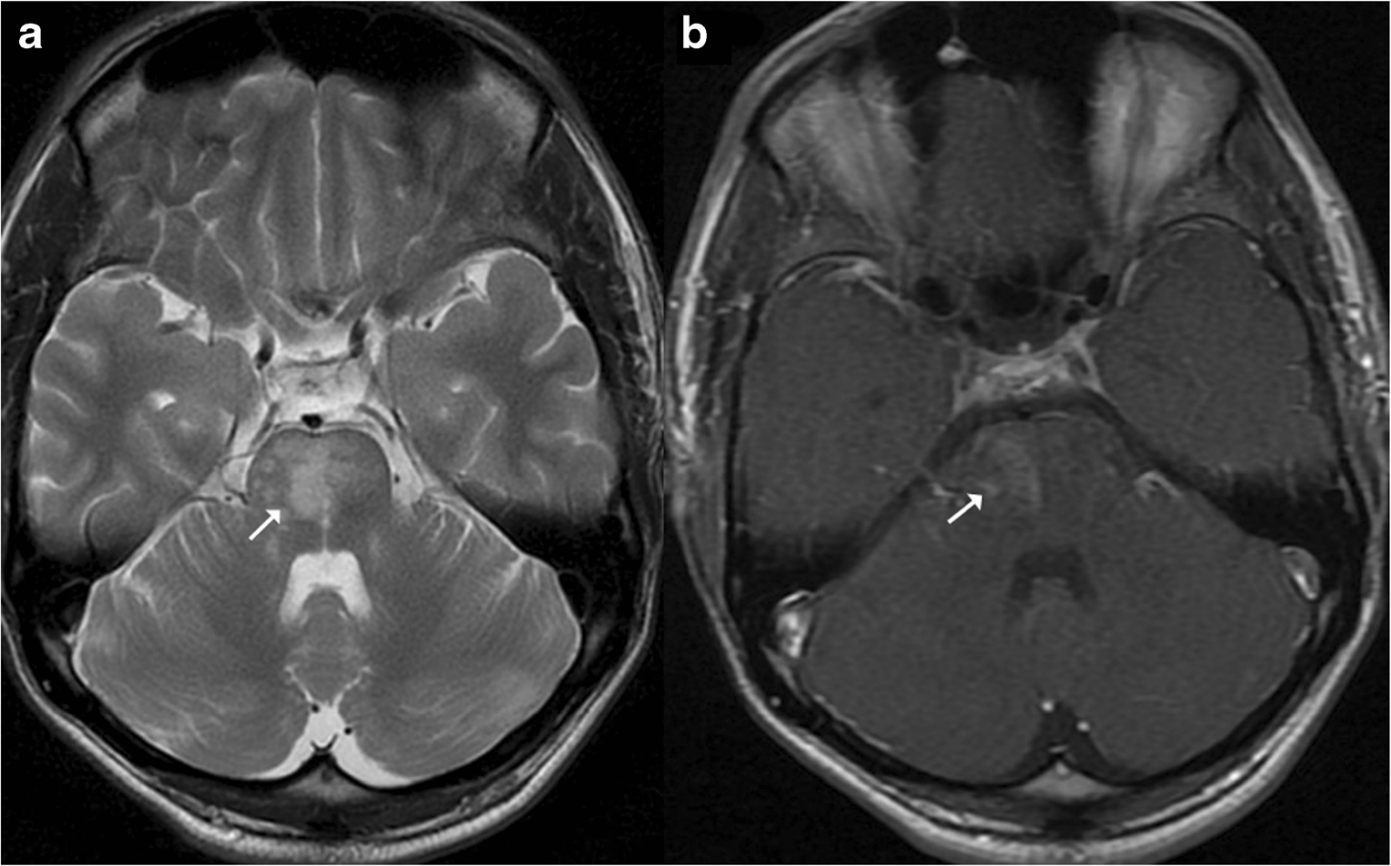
A 16-year-old patient known to have Behçet disease: a Axial T2-weighted image shows increased signal intensity in the pons (*arrow*). b Mild post-contrast enhancement is noted in the involved region (*arrow*)
